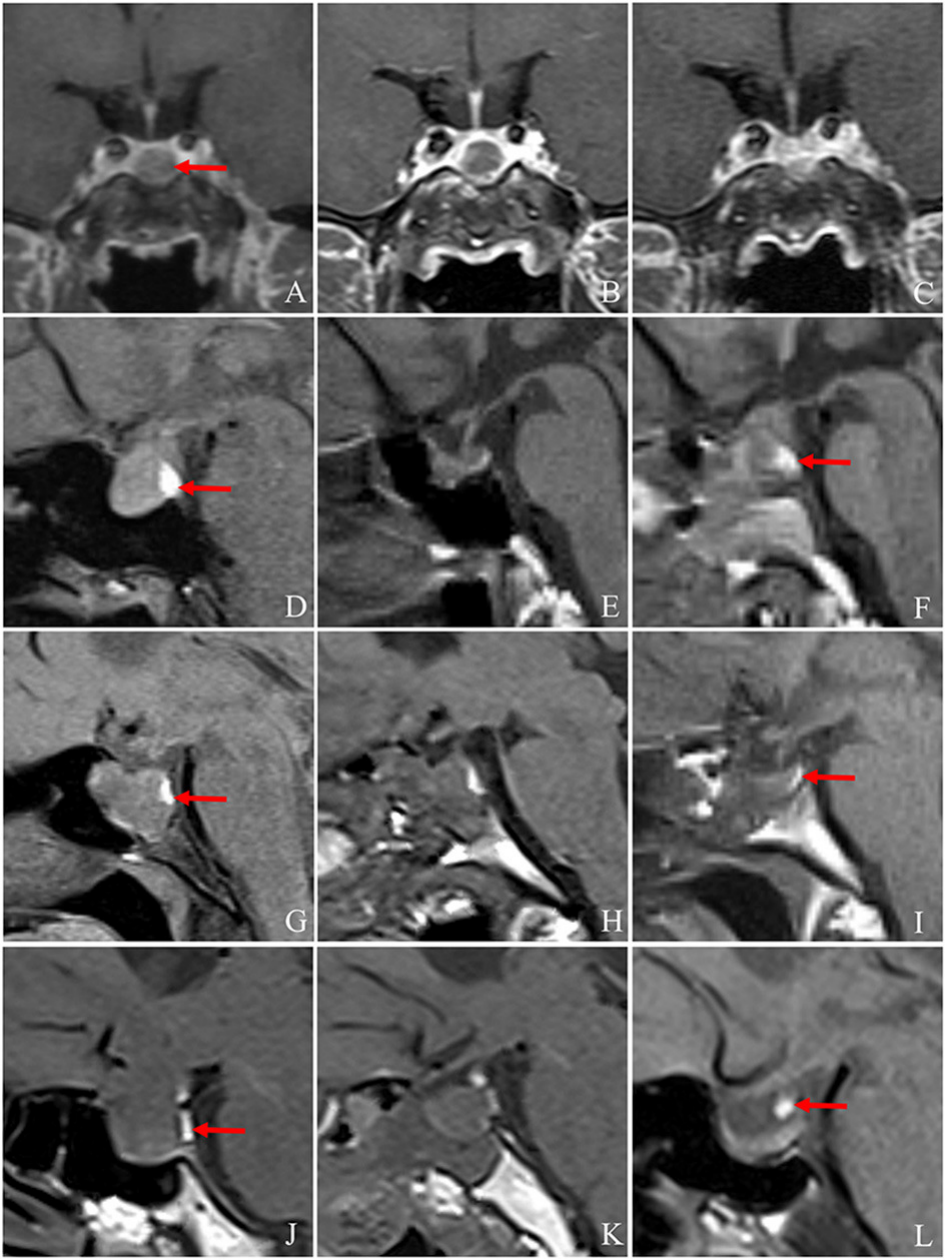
Changes in the pituitary stalk and PPBS pre- and post-operatively. (A) The pituitary adenoma is located directly below the pituitary stalk (arrow) pre-operatively, and the pituitary stalk is centred without deviation. (B, C) MRI showed that the pituitary stalk was in the middle on the 6th day and the 4th month post-operation. (A–C) The pituitary stalk is centred on MRI pre- and post-operatively. (D–F) The PPBS of a 42-year-old female patient persisted pre-operatively and on the third day and at 4 months post-operatively, and the signal intensity decreased slightly post-operatively. The position of the posterior pituitary lobe decreased to the sellar base at 4 months post-operatively, and no obvious abnormality was found in the pituitary stalk (arrow indicates PPBS). (G–I) A 44-year-old female patient with persistent PPBS pre-operatively and on the 1st day and at 4 months post-operatively. The volume of PPBS decreased significantly. The position of the posterior pituitary lobe decreased to the sellar base 4 months post-operatively. No obvious abnormality was found in the pituitary stalk. The patient had no diabetes insipidus post-operatively (arrow indicates PPBS). (J–L) T1WI of a 59-year-old female patient pre-operatively, on the 2nd day post-operatively, and at 4 months post-operatively, respectively. Pre-operatively, two sections of PPBS are observed as being located behind the tumour. Post-operatively, PPBS is located in the lower part of the pituitary stalk, and its volume is larger than that pre-operatively. The patient did not have diabetes insipidus post-operatively (arrow indicates PPBS). MRI, magnetic resonance imaging; PPBS, posterior pituitary bright spots.
